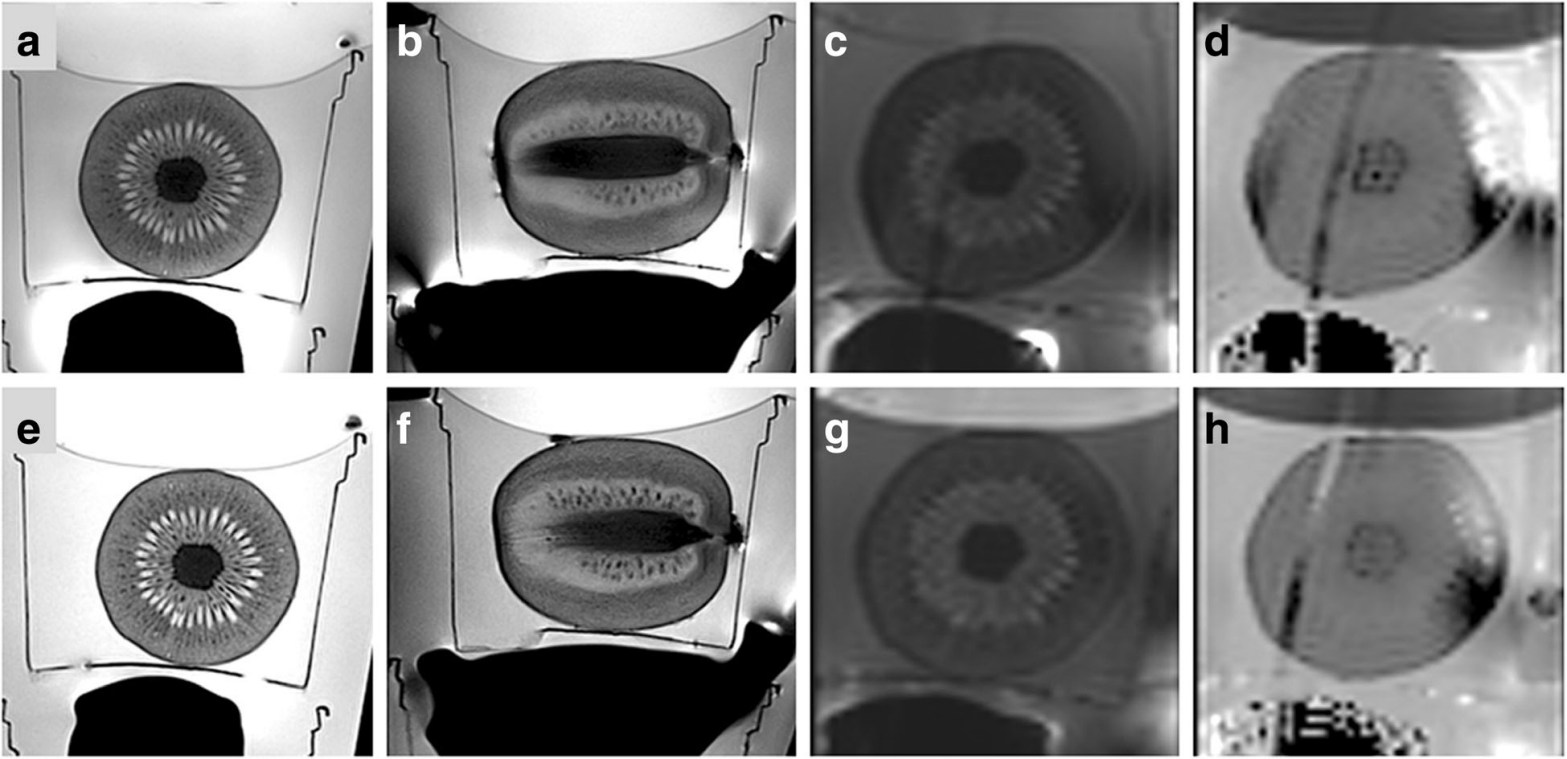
Biparametric magnetic resonance imaging at 3.0 T in a green Hayward kiwifruit (*Actinidia deliciosa*) was performed at site 2 using an endorectal coil included in a multichannel phased-array coil system. Images in the upper panel (a–d) were obtained with air inside the endorectal coil balloon, while images in the lower panel (e–h) were obtained with perfluorocarbon inside the endorectal-coil balloon: axial (a, e) and sagittal (b, f) T2-weighted imaging, axial diffusion-weighted imaging with a *b* value of 800 s/mm^2^ (c, g ), and corresponding apparent diffusion coefficient map (d, h) obtained with a reduced field of view
